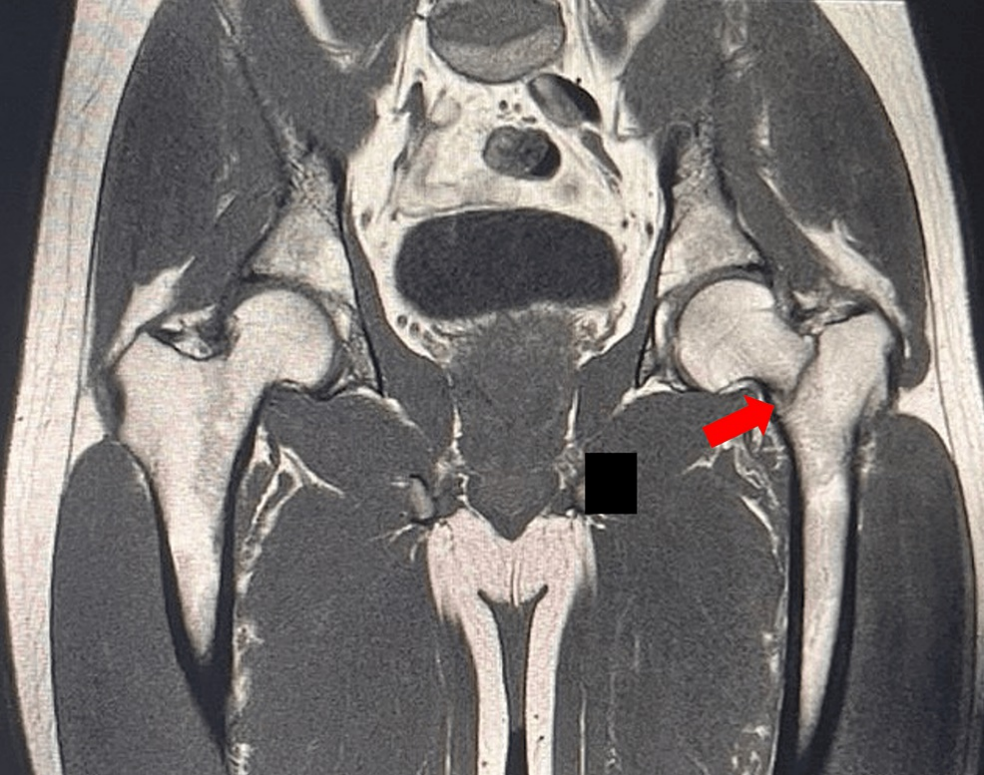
MRI of a Stress Fracture in the Left Femoral Neck MRI image showing a stress fracture of the femoral neck in the left leg, highlighted by a red arrow. A black box is placed over the radiologist's cursor to prevent distraction.
